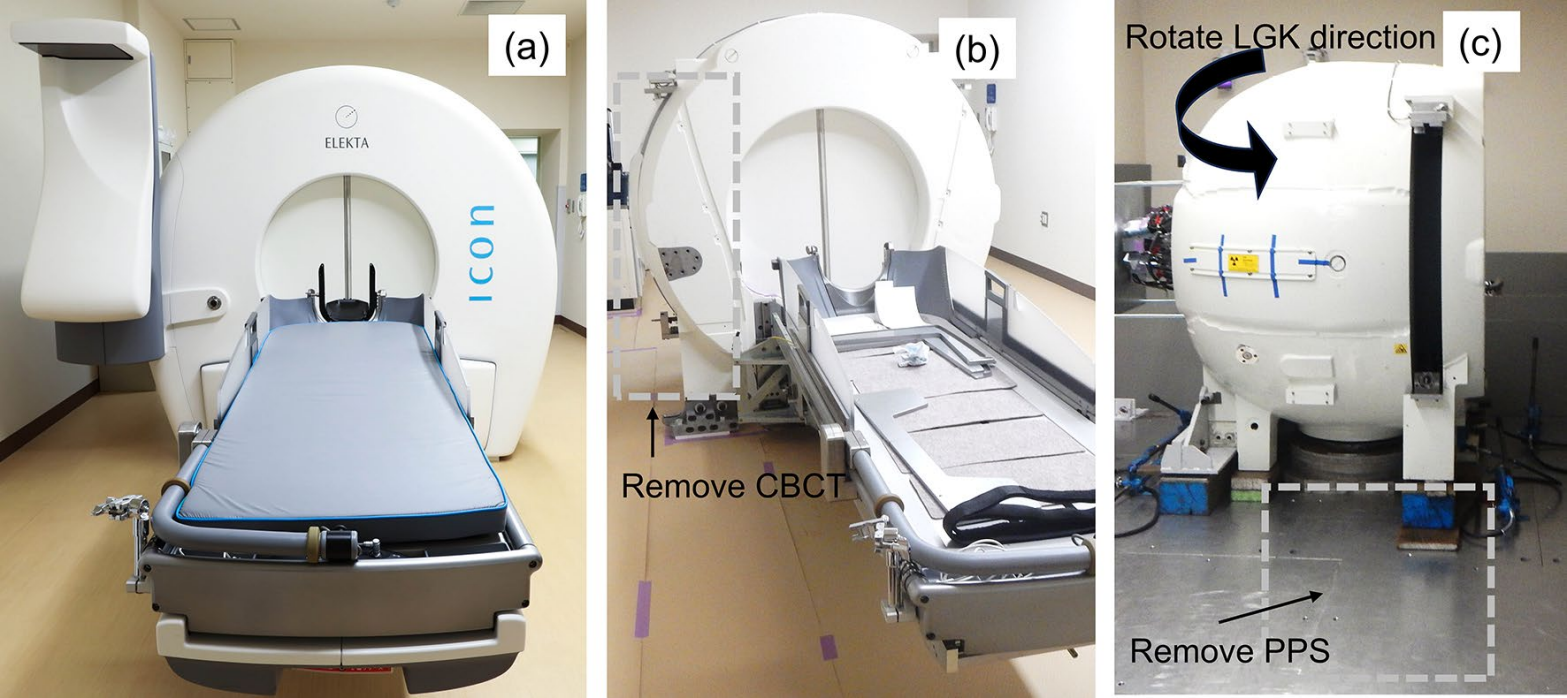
a Overview of the LGK system, and b the source replacement extensive work to remove the CBCT, and c PPS and rotate the LGK unit
